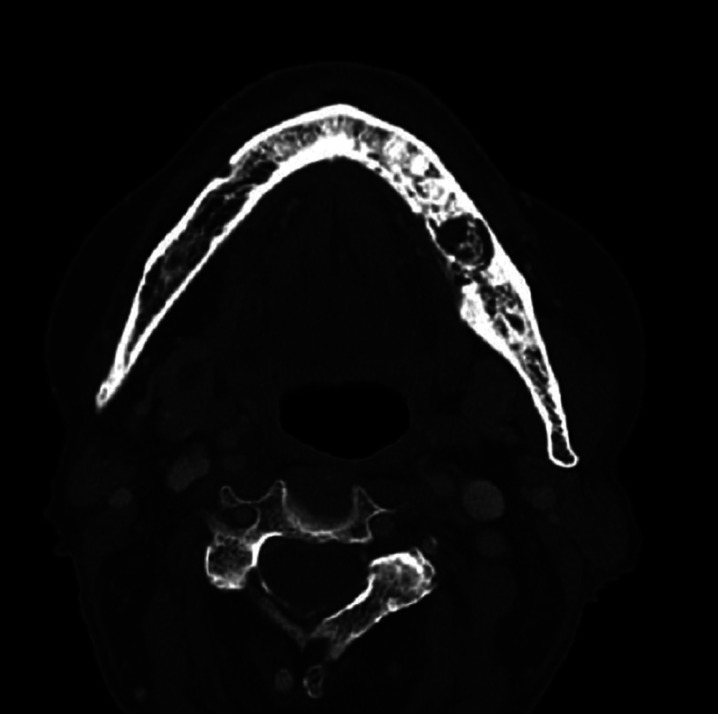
Computed tomography with evident mandibular osteolysis in case 6.
